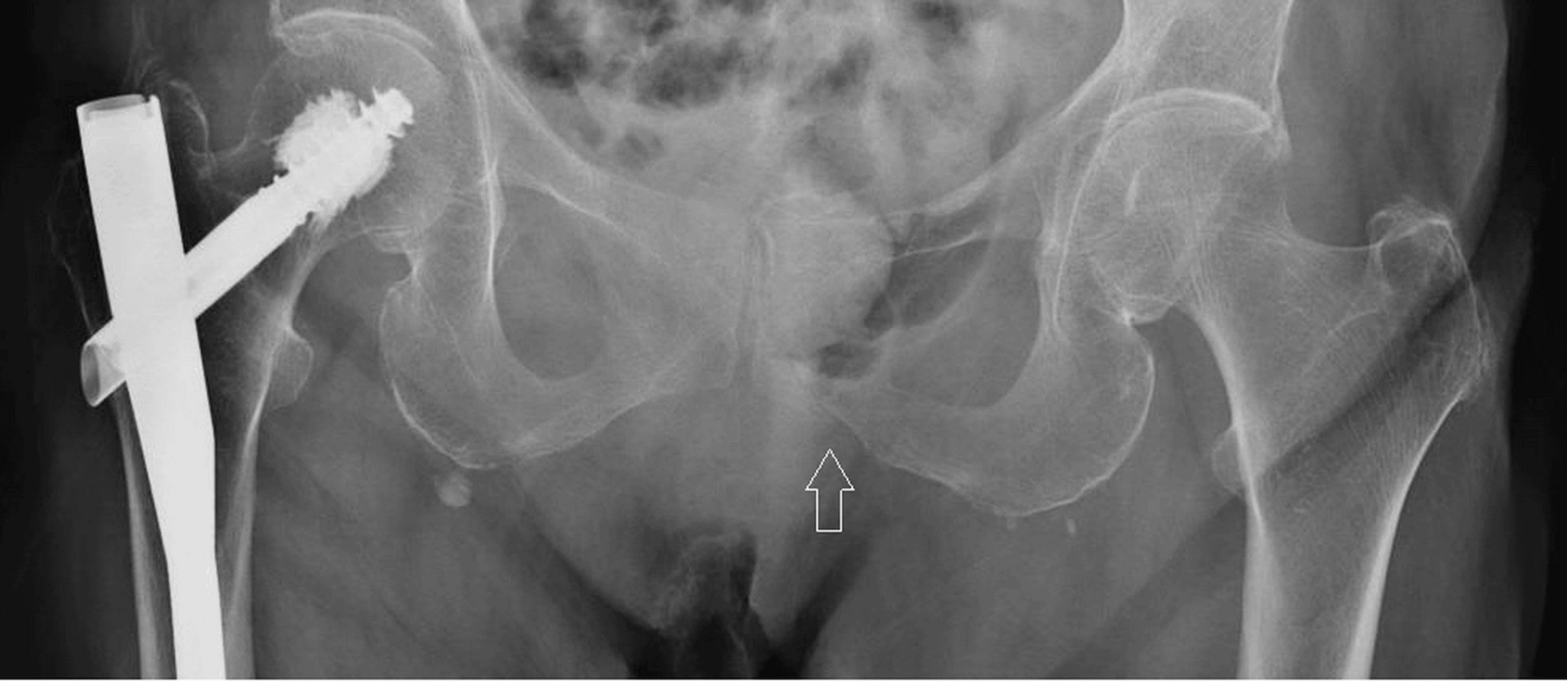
Pelvic radiograph demonstrating the left inferior pubic ramus fracture. Anteroposterior pelvic radiograph shows subtle cortical disruption of the left inferior pubic ramus (arrow), suspicious for a nondisplaced fracture. Prior fixation hardware in the right proximal femur is also visualized.
